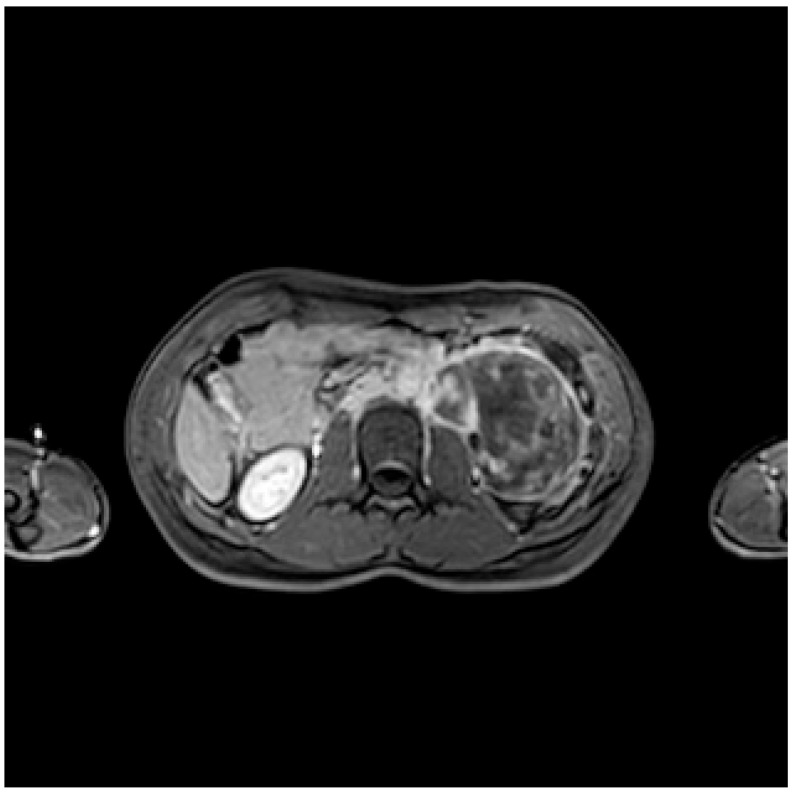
The T2-weighted fat-saturated sequence shows a hyperintense signal of the lesion with a medial hypointense dishomogeneity.
